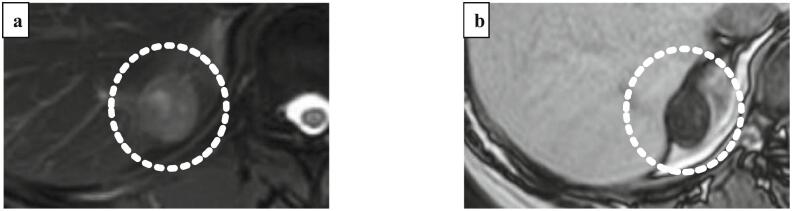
(a) On magnetic resonance imaging (MRI), T2-weighted fat-suppressed imaging showed that there was high intensity at the tumor, (b) chemical shift imaging showed markedly low intensity at the tumor (indicated by white dotted circle).
